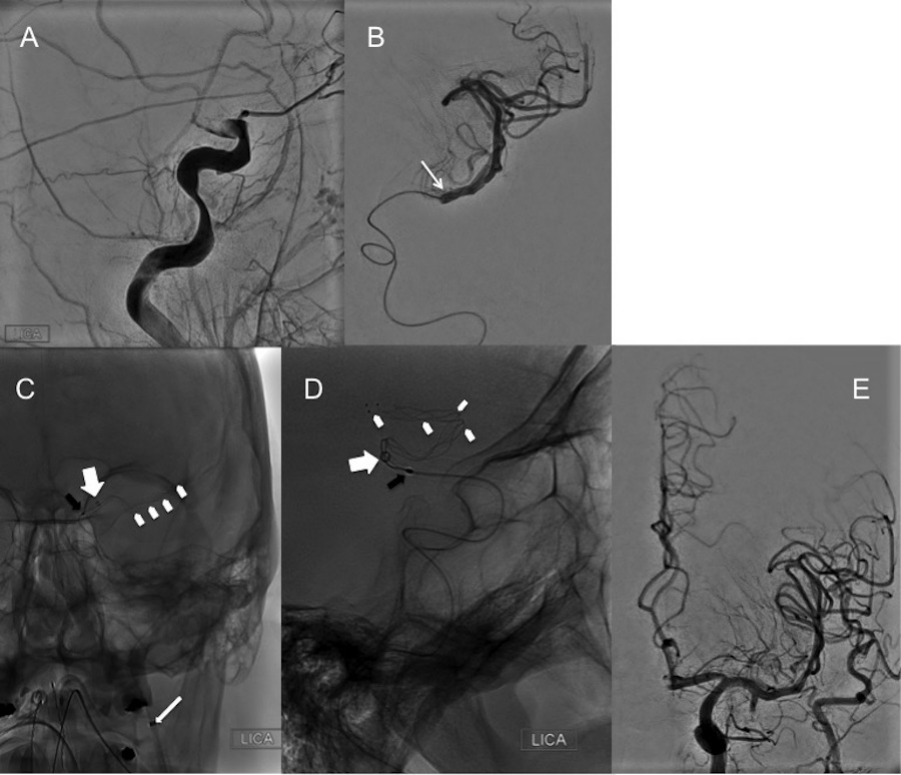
Case 1 (A) Magnified lateral oblique view of the high cervical and intracranial left ICA. There is no antegrade progression of contrast beyond the ophthalmic segment of the left ICA (white arrow). (B) Frontal DSA run through a microcatheter positioned distal to the clot in the left M1. (C) and (D) are frontal and lateral magnified radiographs moments prior to the thrombectomy; the BGC (long white arrow) has not yet been inflated and is distal to the recently placed cervical ICA stent, the tip of the Arc catheter is in the proximal left M1 (short white arrow), the microcatheter has been retracted following the PFT (tip is inside the Arc catheter, small black arrow), and the stent-retriever has been exposed (white arrowheads). (E) Final DSA run with a frontal view of the head demonstrates TICI 2B outcomes with residual occlusion of the left anterior temporal artery (long white arrow). LICA = Left internal carotid artery.
